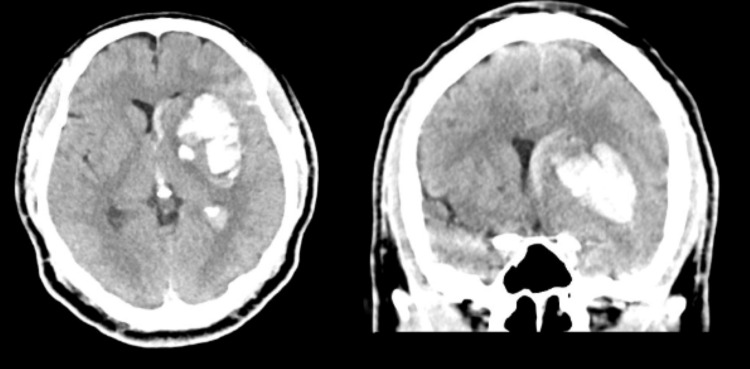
Preoperative CT scan for patient 1 CT scan with a left basal ganglia intracerebral hemorrhage (ICH)
